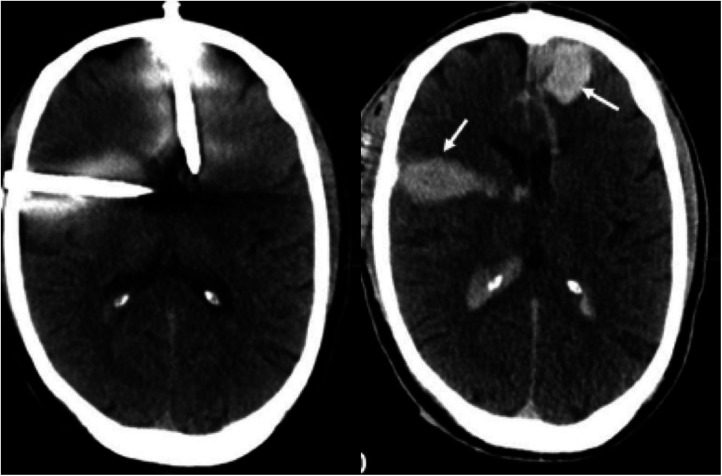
Cranial CT-scan before removal of the nails from the skull (left) with discrete bleeding and after removal with a progressive hematoma (arrow) (right).
